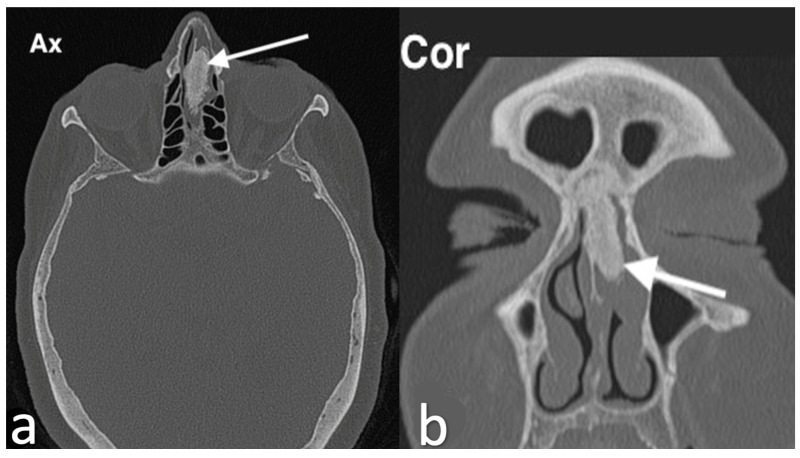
Osteoma as an incidental finding in a patient with rhinosinusitis. CT shows, in the upper anterior third of the nasal septum, an oval mass with smooth margin and bone density (arrow) visible on axial (a) and coronal (b) images. The mass extends into the left nasal cavity, partially obstructing the frontal recess.
